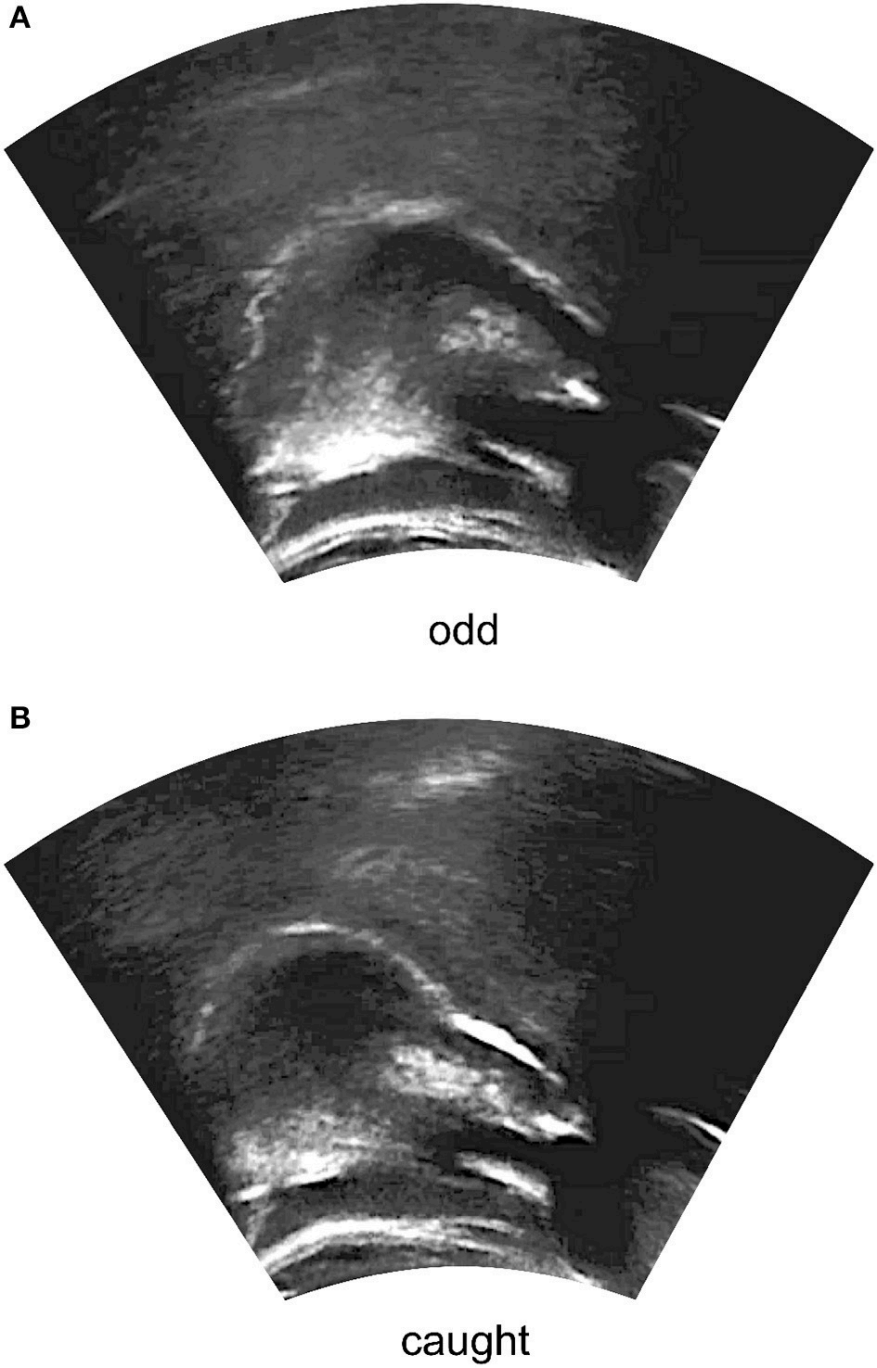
Extracted midsagittal ultrasound frames for the tokens (A) odd and (B) caught, as produced by Speaker 6. The right side of the image corresponds to the front of the mouth. The tongue surface is visible as the white line near the center of the image. The dark portions on the left and right sides of the image are the shadows of the hyoid and the mandible.
